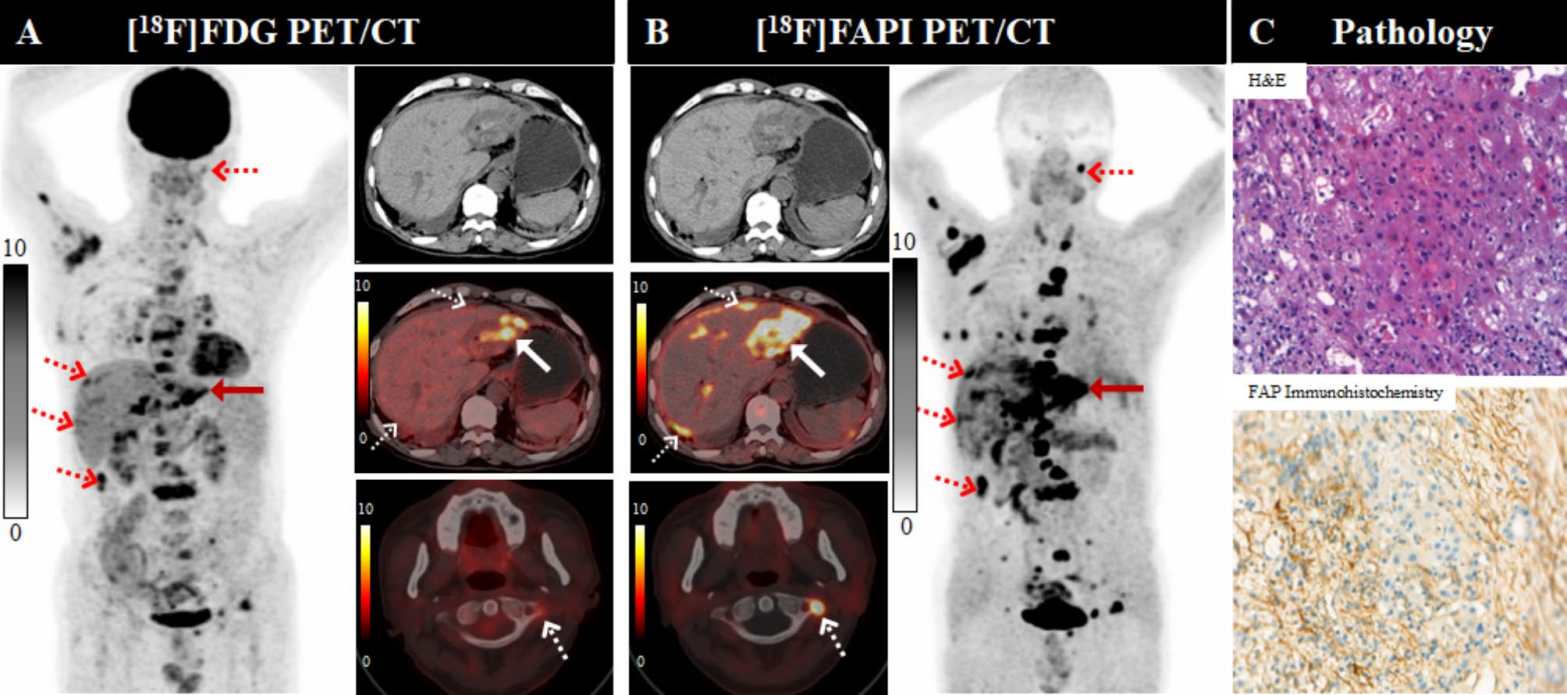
A 61-year-old male patient (Patient No. 16) with intrahepatic cholangiocarcinoma (ICC) was confirmed by biopsy. Compared with [^18^F]FDG PET/CT) (A), [^18^F]FAPI-04 PET/CT (B) demonstrated higher radiotracer uptake in the primary tumor ( SUV_max_ of 13.7, solid arrow), as well as in peritoneal and bone metastatic lesions (dotted arrows). Pathological findings (C) indicate a heightened expression for FAP
